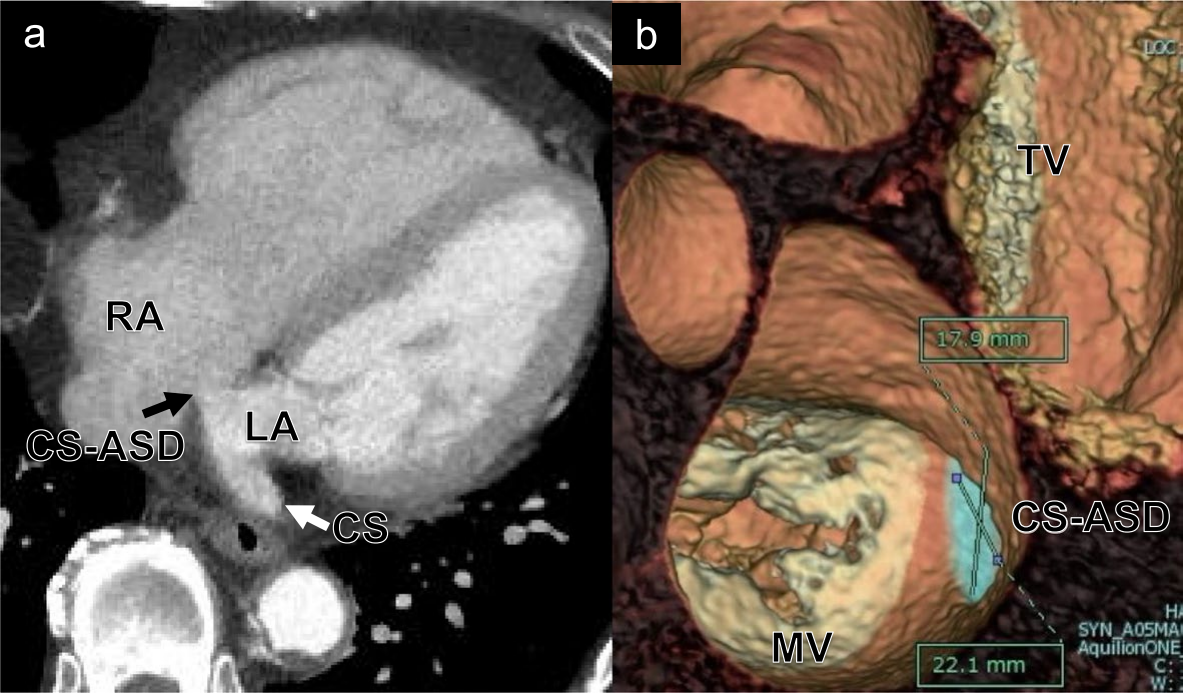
Enhanced CT showed a communication between the left atrium and CS (a). 3D reconstruction CT revealed that the defect was located adjacent to the mitral valve annulus, measuring approximately 18 mm × 22 mm (b). LA, left atrium; RA, right atrium; CS, coronary sinus; CS-ASD, coronary sinus atrial septal defect; MV, mitral valve; TV, tricuspid valve; CT, computed tomography; 3D, three dimensional
